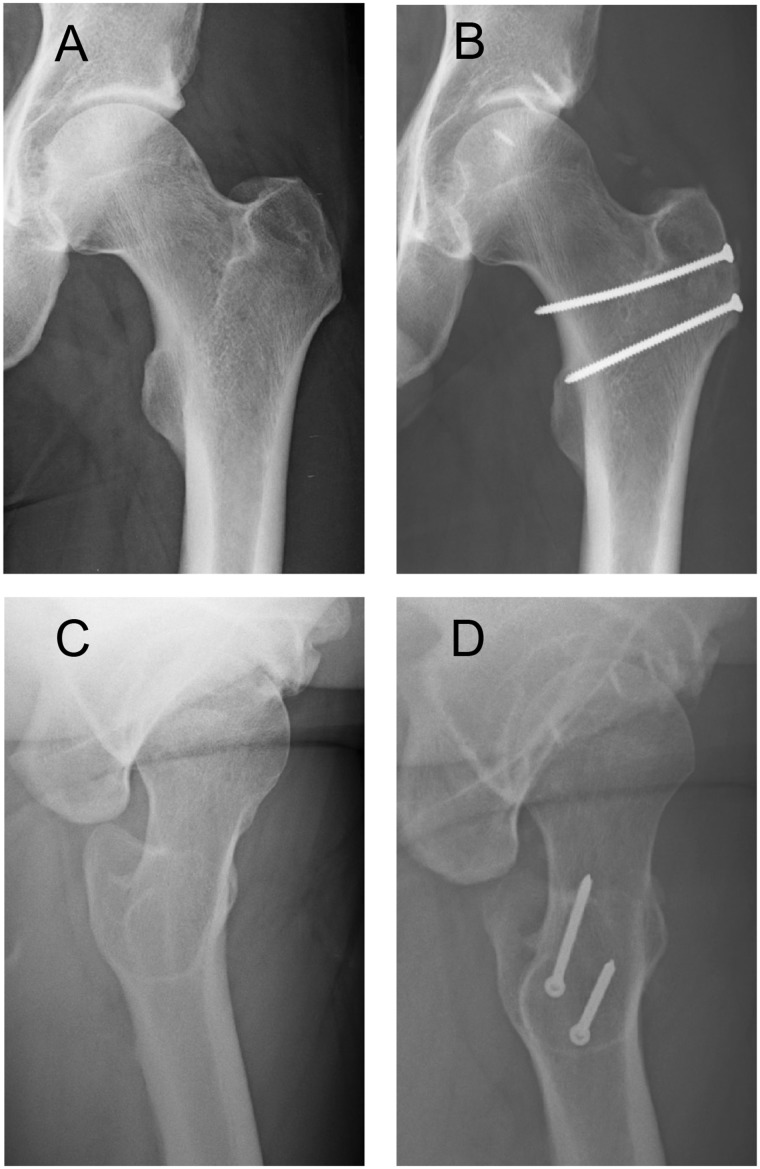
Pre- (A, C) and postoperative (B, D) X-ray of the left hip of Patient Number 2. (A) Preoperative X-ray in a/p pelvic view with an LCE of 39°, and (B) corrected LCE of 31° postoperatively. (C) Alpha angle of 62° preoperatively was corrected to (D) 44° postoperatively in the lateral cross table radiograph. Acetabular labrum was fixed with four bone anchors from.
